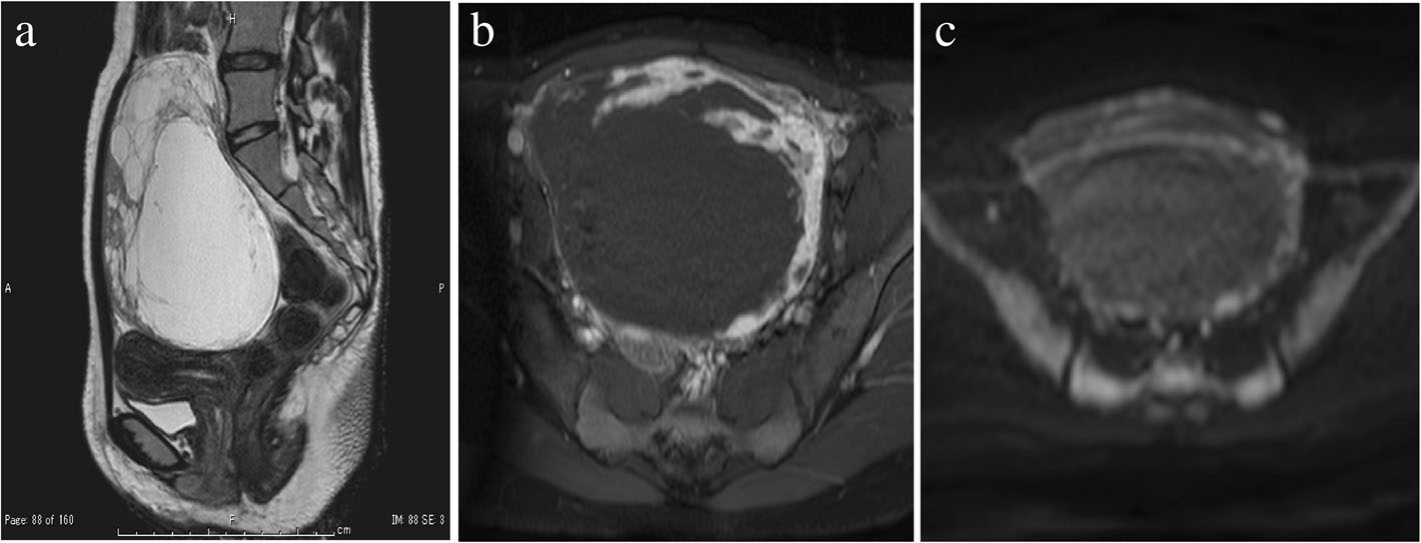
a. On sagittal T2-weighted imaging (repetition time [TR]/echo time [TE]: 1500/147.39 ms), the cystic components have the same signal intensity (SI) as water, and the irregularly thickened septa and solid components (arrows) have intermediate SI, higher than the SI of uterine myometrium. b. On axial contrast-enhanced T1-weighted imaging (repetition time [TR]/echo time [TE]: 534/13 ms), the septa and solid components (arrows) show early strong enhancement. c. On axial diffusion-weighted imaging (TR/TE: 1950/70 ms, b = 1000 s/mm2), the septa and solid components show slight hyperintensity
